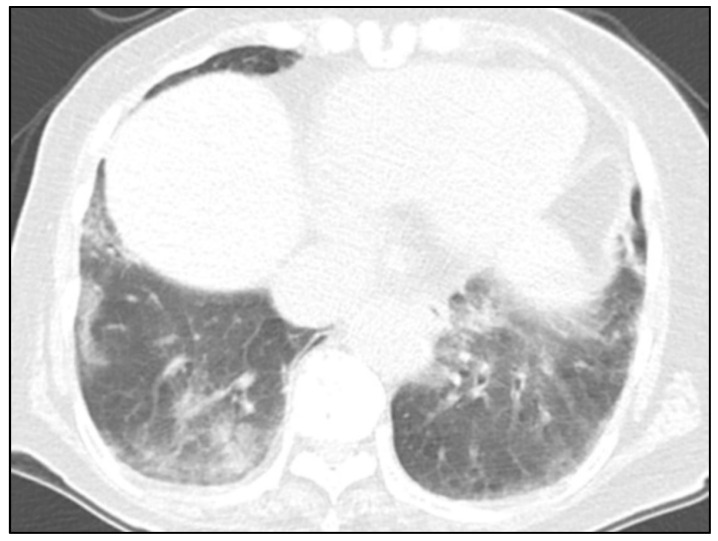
Chest CT image in lung window setting showing patchy consolidation and ground-glass opacities in both lower lobes.
